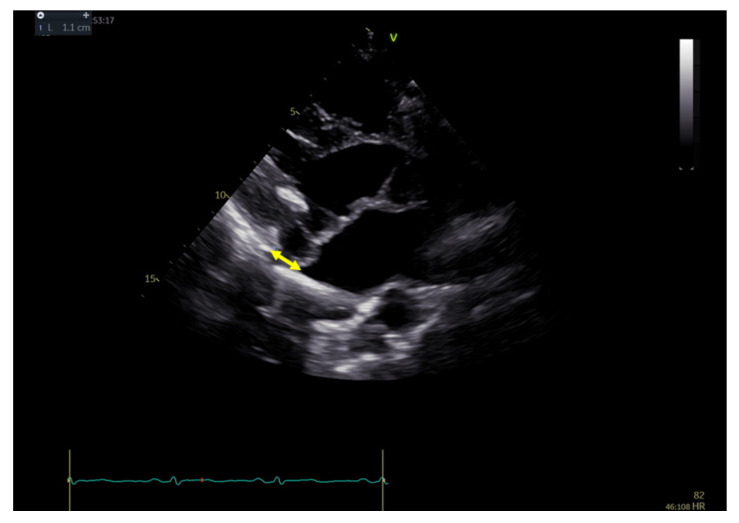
Transthoracic echocardiography (long-axis parasternal view) of MAD measurement in MAD+ patients. MAD distance is calculated as the length of systolic separation between the left atrial wall and mitral valve leaflet junction to the top of the left ventricle free wall.
